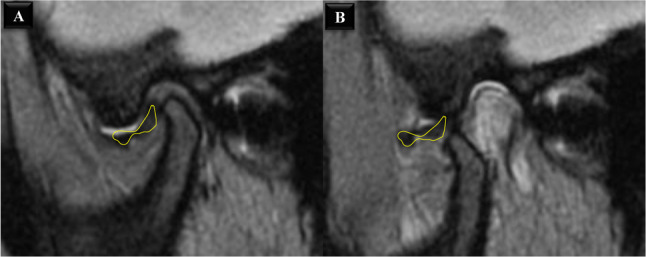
MRI showing DDwoR: (A) MRI in closed mouth position showing articular disc displaced anteriorly (outlined in yellow), (B) MRI in closed mouth position showing that the articular disc is still anteriorly displaced confirming DDwoR (outlined in yellow)
